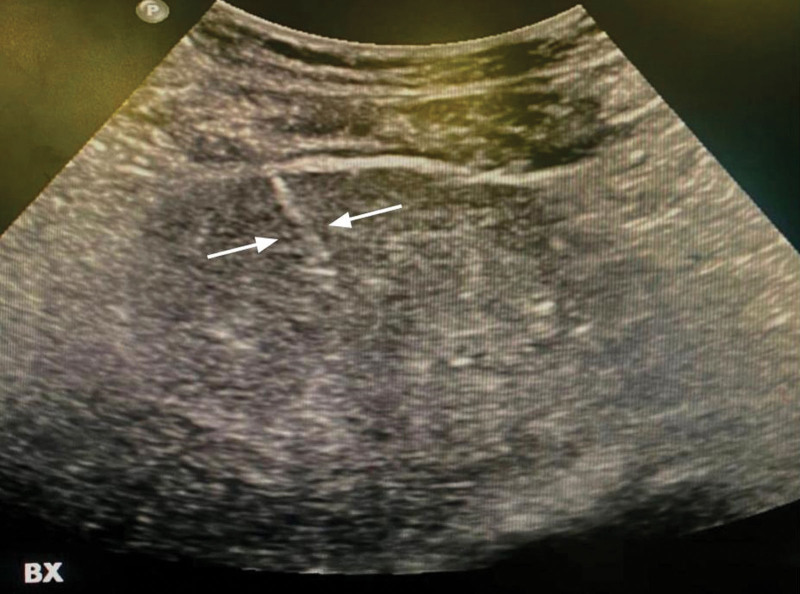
Frozen ultrasound image of the needle (white arrow) in the liver.
